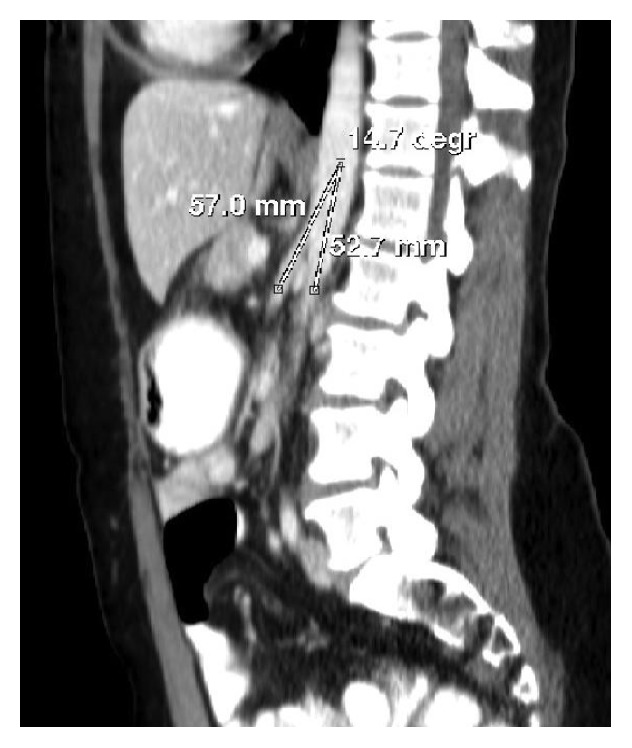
CT sagittal view showing an angle of 14.7 degrees between the SMA and aorta (Case Report Two).
